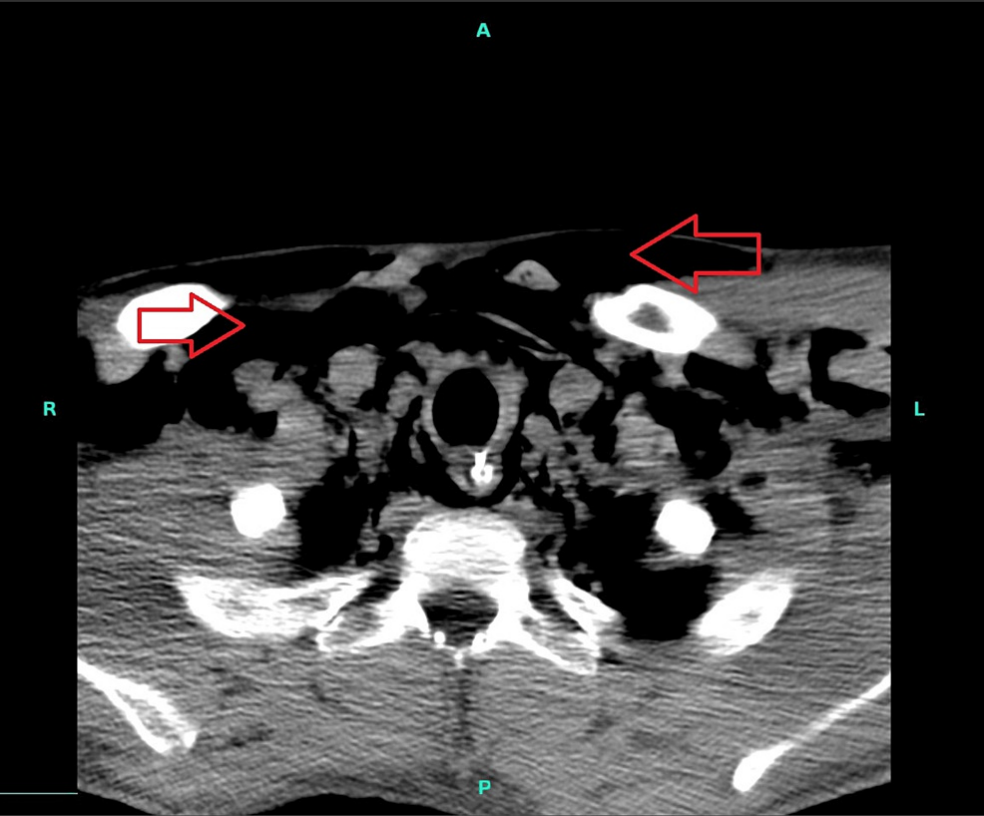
CT chest without intravenous contrast showing subcutaneous emphysema throughout the bilateral neck and upper chest wall (red arrows)
